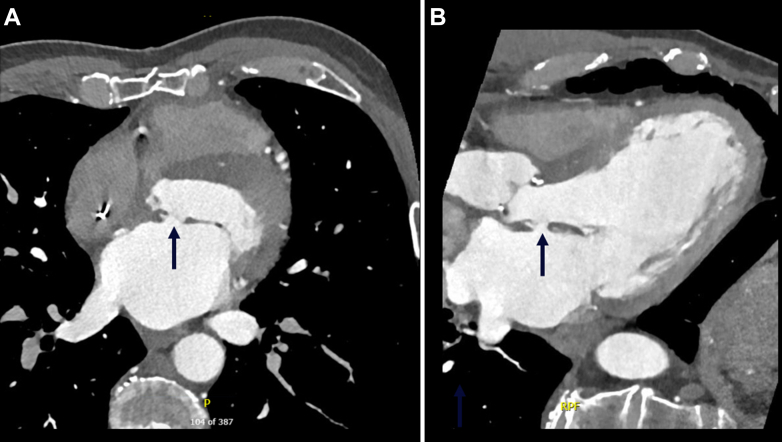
Preprocedural Cardiac Computed Tomography (A) Axial plane and (B) sagittal plane computed tomography reveal a 10 × 10 mm defect (arrows) of the basal anterior mitral valve leaflet, despite patch repair 1 year prior.
